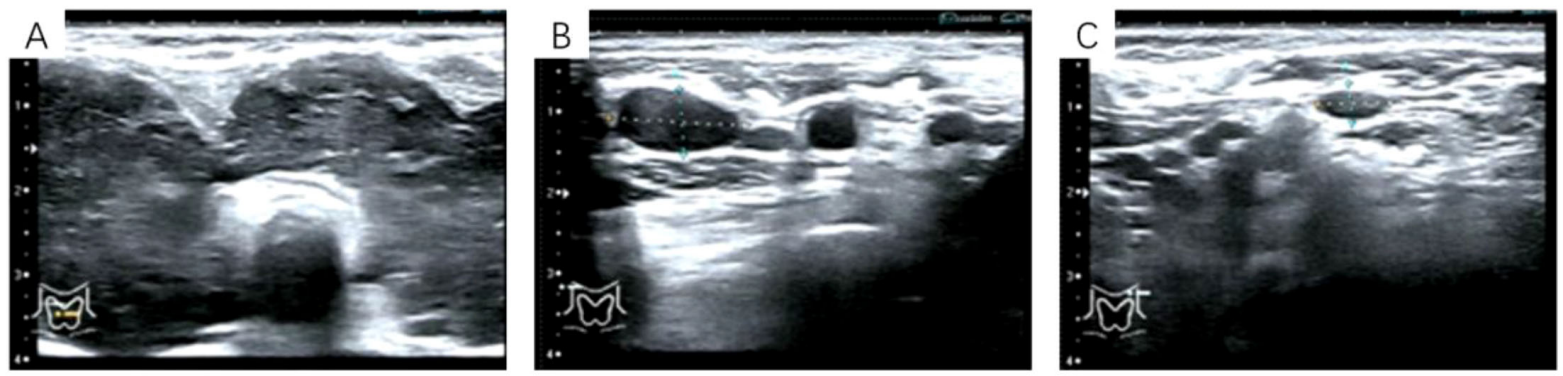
(A) Color Doppler ultrasonography of the thyroid. (B) Ultrasonography of the right level III cervical lymph node. Dashed lines indicate the dimensions: 9.8 mm (longitudinal) × 4.7 mm (transverse). (C) Ultrasonography of the left level III cervical lymph node. Dashed lines indicate the dimensions: 10.3 mm (longitudinal) × 7.5 mm (transverse).
